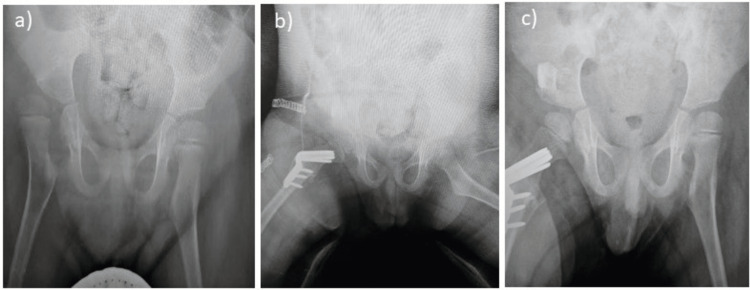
Plain x-rays of a six-year-old patient with right hip dislocation (a) pre-operative x-ray; (b) x-ray of the same patient immediately after surgery with reduced right hip; (c) x-ray of the same patient at the 20-months follow-up showing a reduced hip joint.
